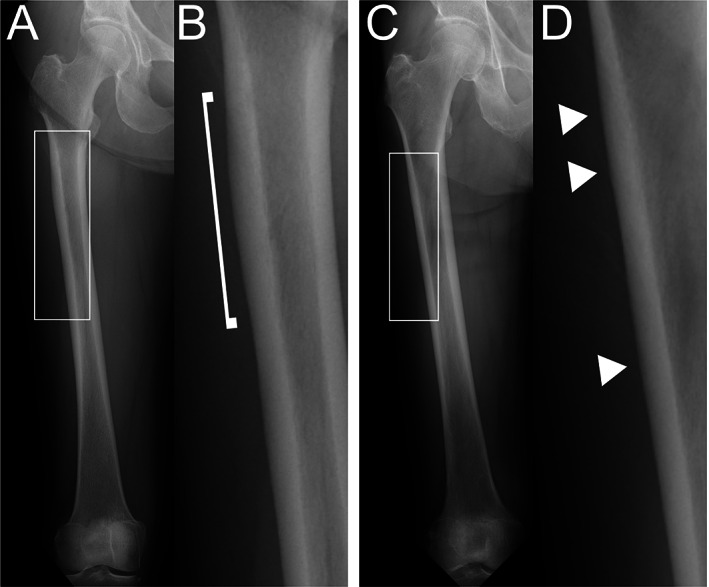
Images for two patients with grade 1 radiographic findings. (A, B) Images for first patient, a 72‐year‐old woman with bone metastasis from breast cancer who had received oncologic denosumab for 13 months. (A) Anteroposterior image of right femur. (B) Enlarged image of white box in Fig 5A shows diffuse thickening of subtrochanteric lateral cortex in right femur (open square bracket). She was assessed as grade 1. (C, D) Images for second patient, a 77‐year‐old man with bone metastasis from hepatocellular carcinoma who had received oncologic denosumab for 40 months. (C) Anteroposterior image of right femur. (D) Enlarged image of white box in Fig 5C shows minute local thickenings of subtrochanteric and midshaft lateral cortex in right femur (arrowheads, maximum TR <1.1). This patient was also assessed as grade 1. TR, thickening ratio.
